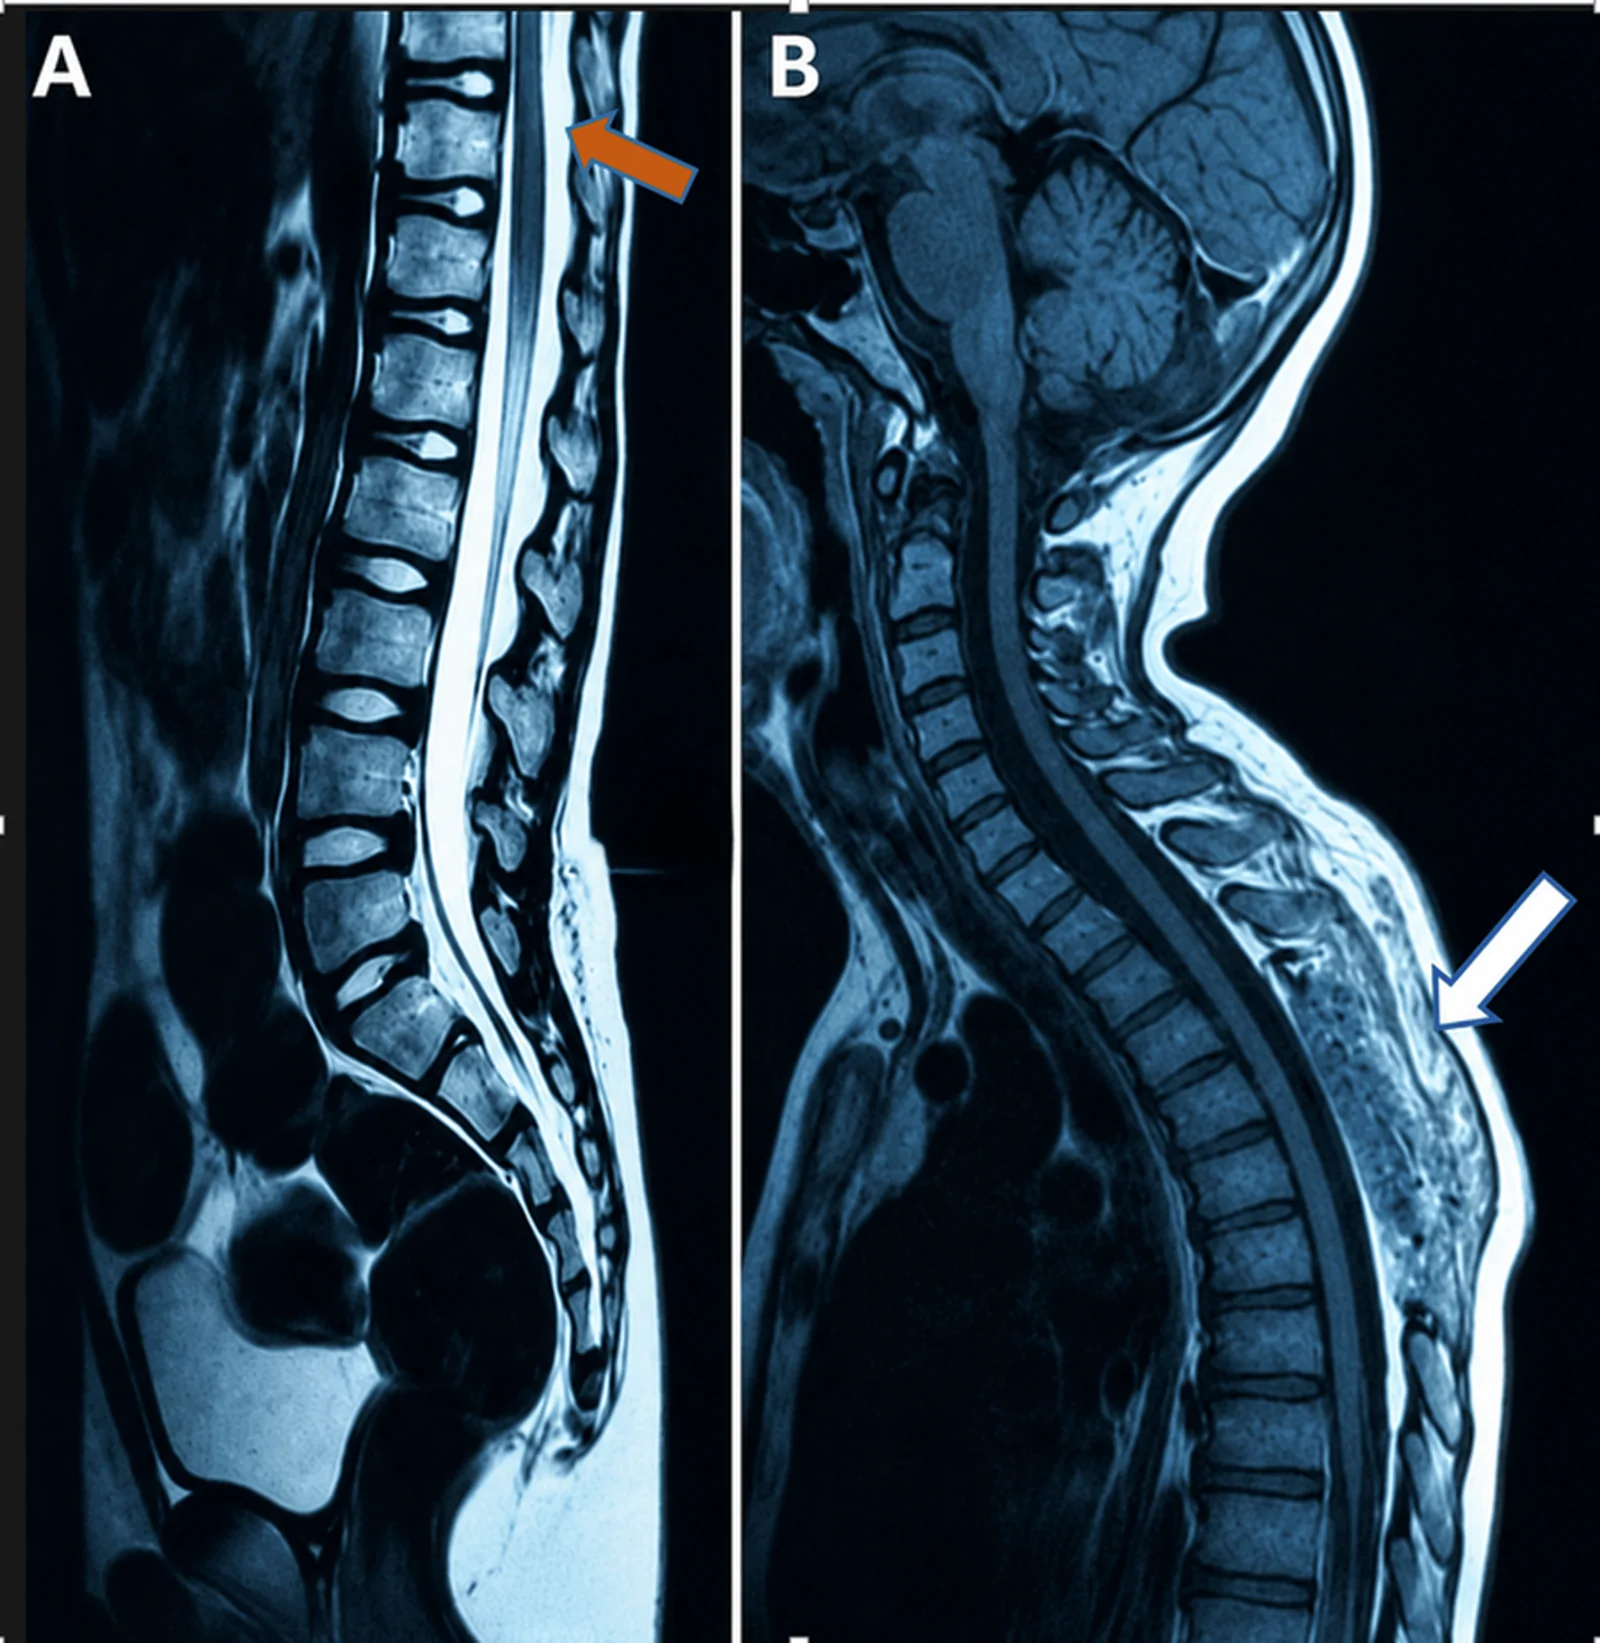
Postoperative spinal MRI A: T2-weighted thoracic spinal MRI showing complete disappearance of the posterior epidural lesion (brown arrow) after laminectomy, with resolution of spinal cord compression.
